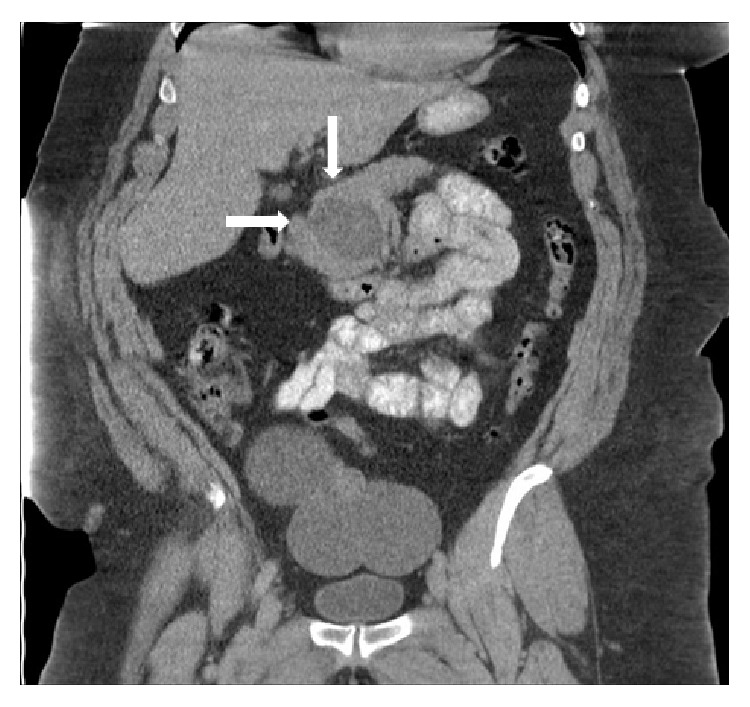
MRI of a patient with autoimmune pancreatitis in coronal orientation showing a tumor in the pancreatic head (focal type; white arrows).
